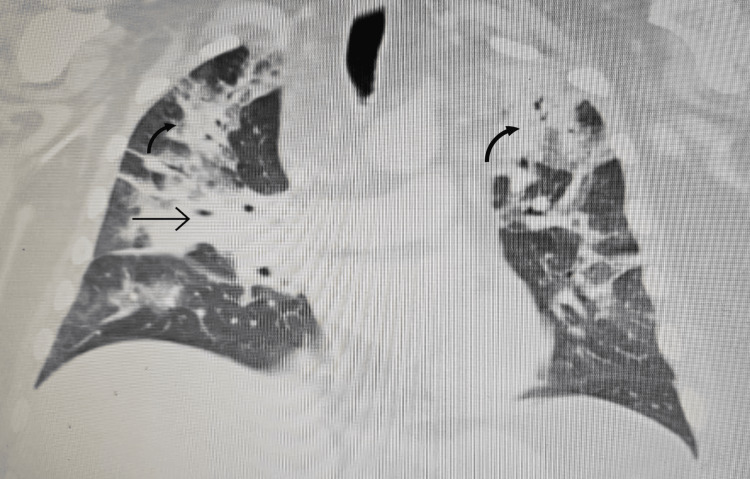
A chest X-ray revealing (arrows) bilateral multilobar pneumonia
